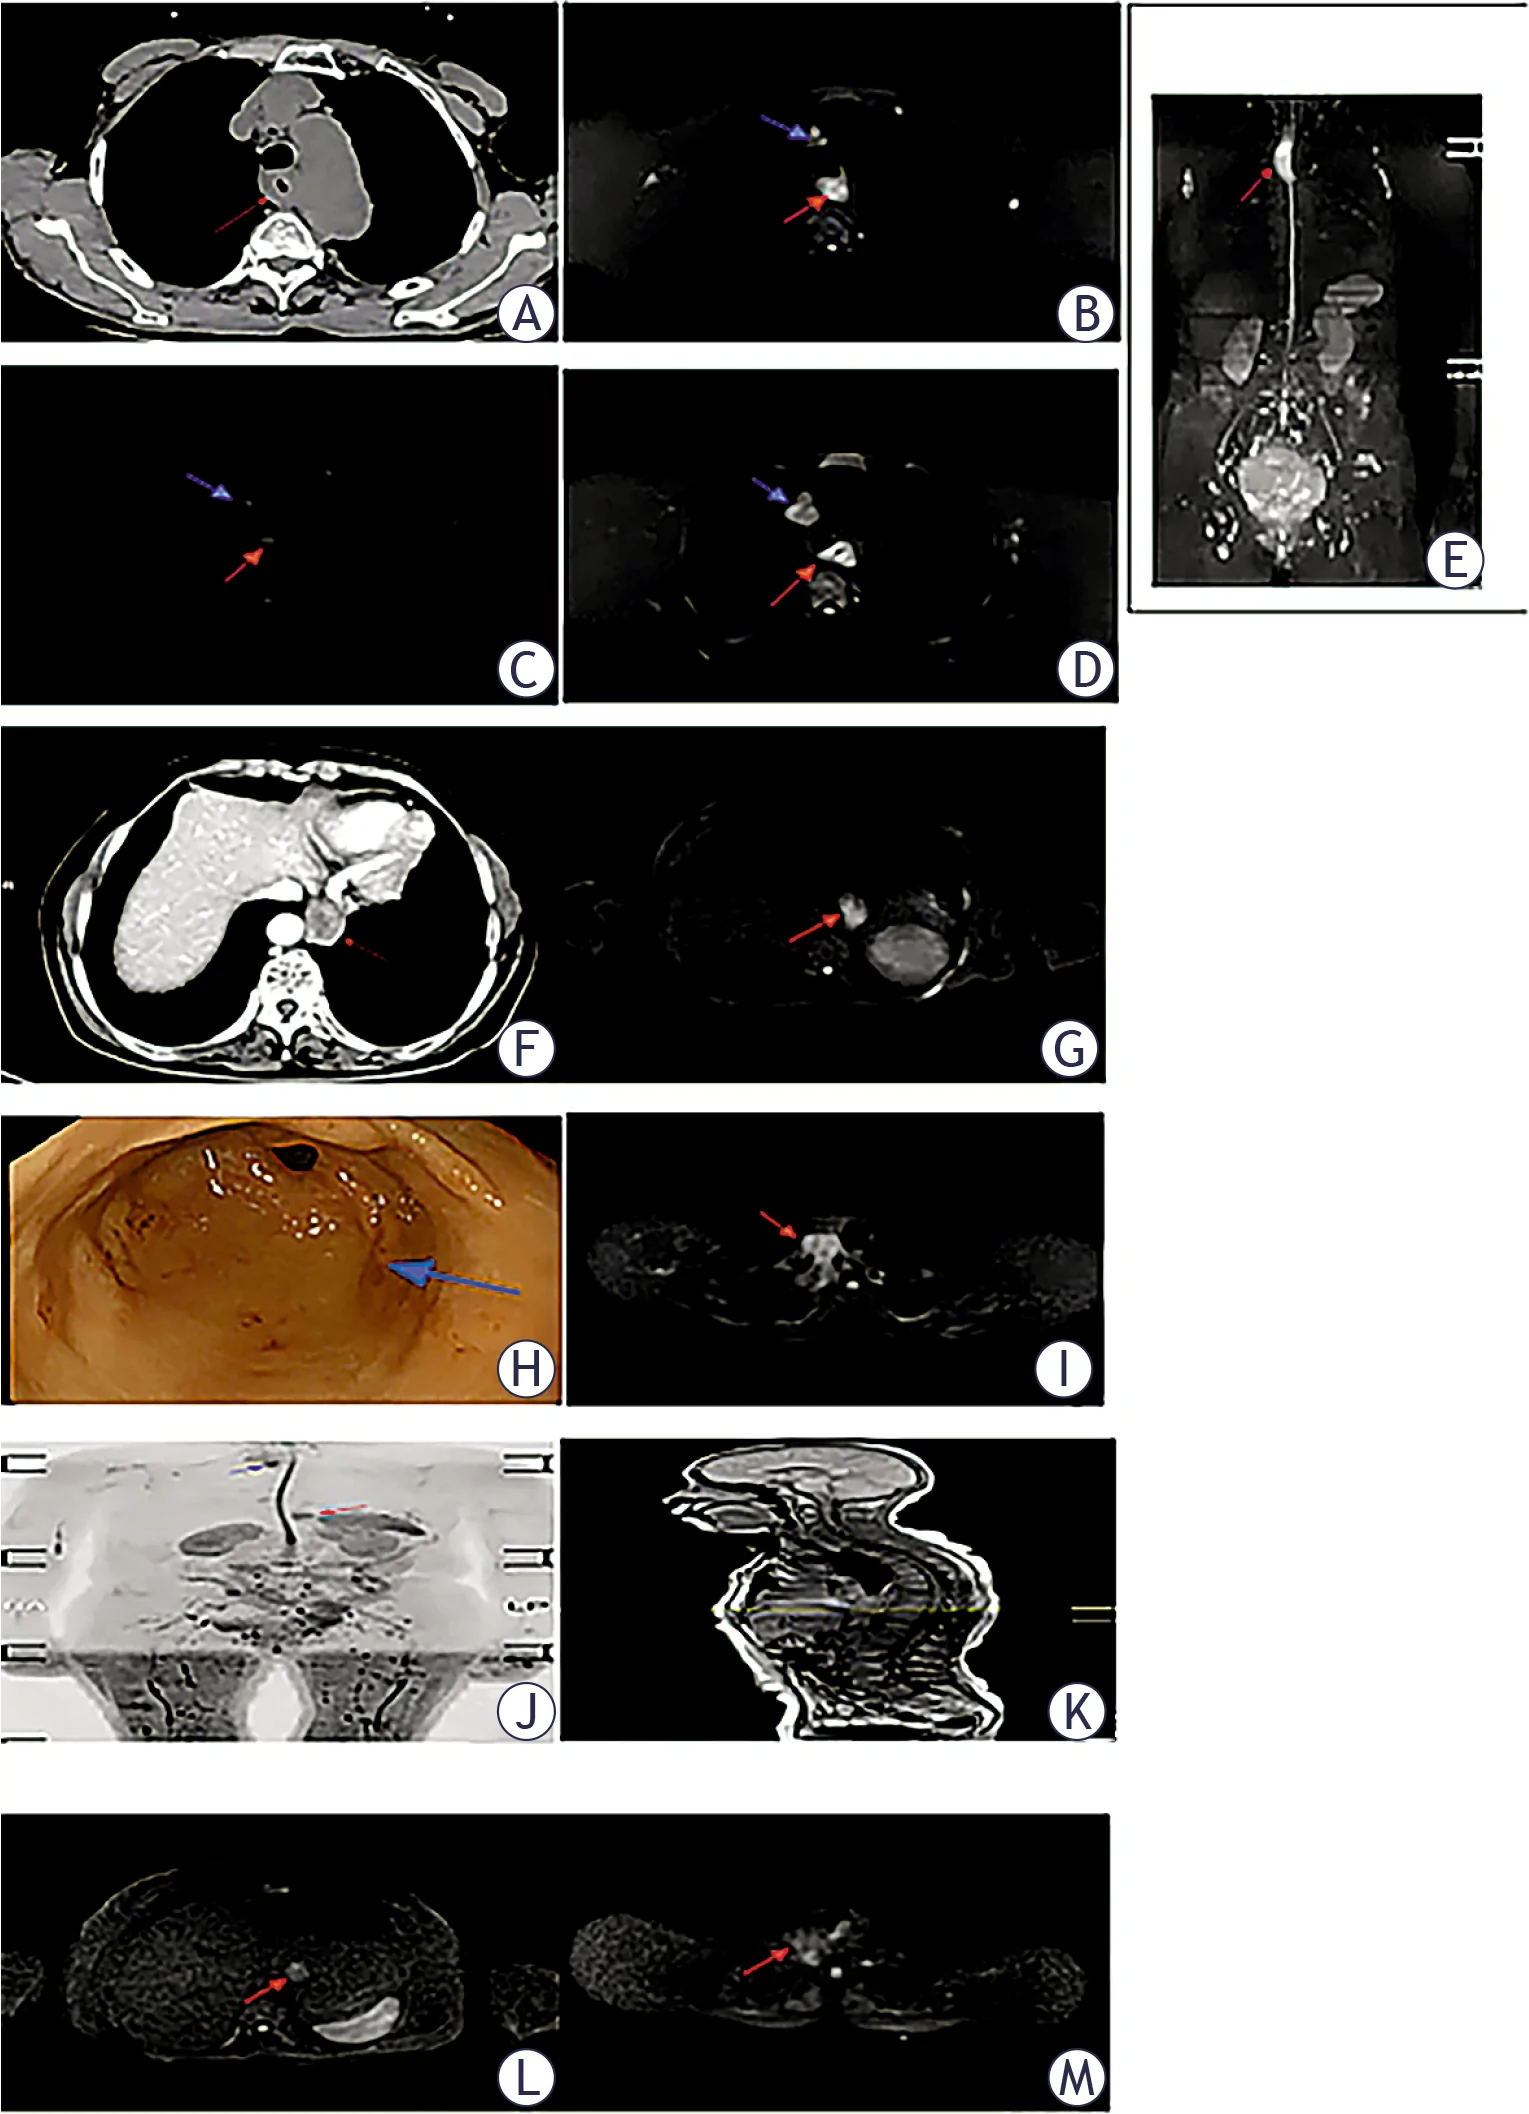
Serial whole-body DWI for dynamic treatment monitoring and restaging in two representative esophageal cancer patients. *Case 1*
